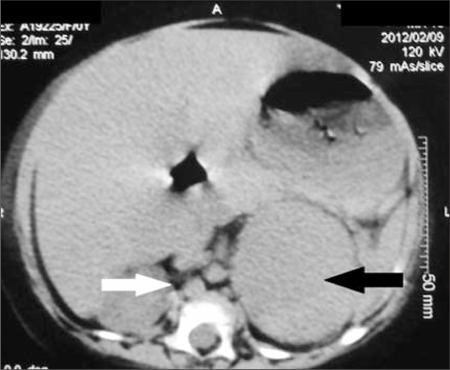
CT of abdomen showing homogenous left adrenal mass with distinct borders (black arrow); contra-lateral adrenal could not be visualized (white arrow)
